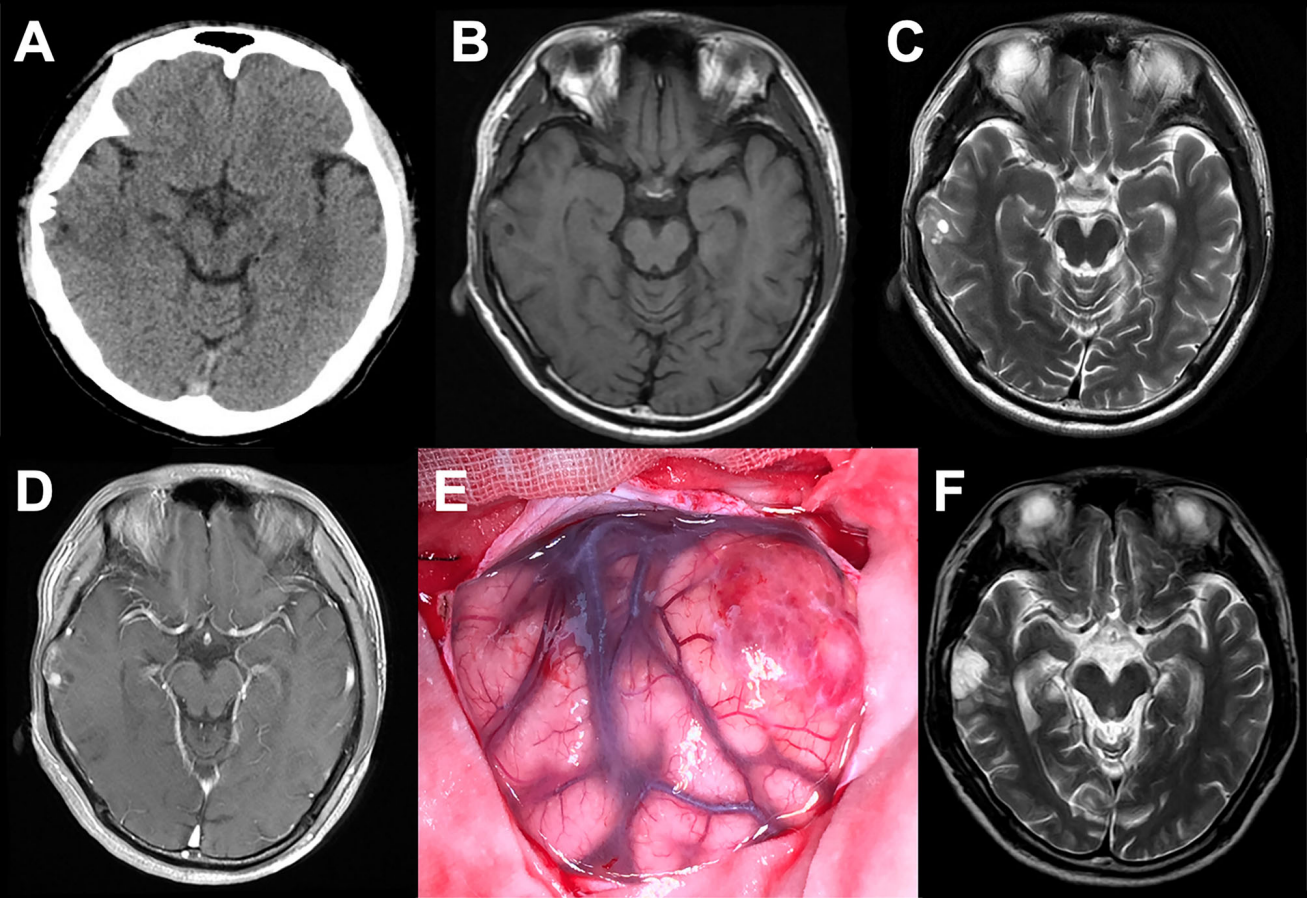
Data of case c. (A) Axial CT showing a superficial situated mass in the right temporal lobe with patchy calcification (B–D). Preoperative MR scans. The mass displayed a heterogeneous signal with cystic components, and a hypointense signal on T1WI, a hyperintense signal on T2WI, and partial enhancement on T1W1 post-gadolinium imaging. (E) Operative view before tumor resection. (F) Postoperative MRI showing the gross-total resection. The patient was seizure-free for 22 months.
